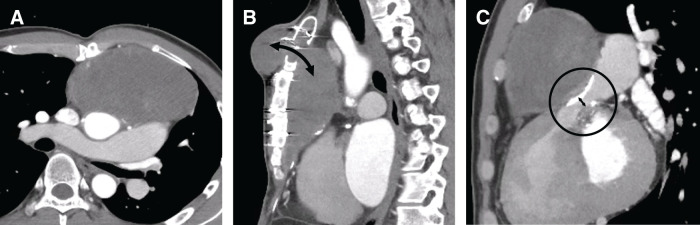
Preoperative CT images of chronic expanding hematoma. (A) Axial image demonstrating a mediastinal mass measuring 110 × 65 mm. (B) Sagittal image showing continuity between the subcutaneous and mediastinal masses through an osteolytic defect in the manubrium (black arrow). (C) The mediastinal mass compressing the right ventricular outflow tract (circle), with narrowing to 9 mm in diameter (double-headed arrow).
